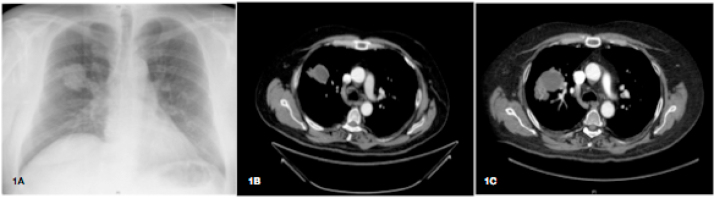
1A: Chest X-ray on admission. 1B: Chest CT scan on admission. 1C: Chest CT scan on day +100, with no response to antifungal therapy.
